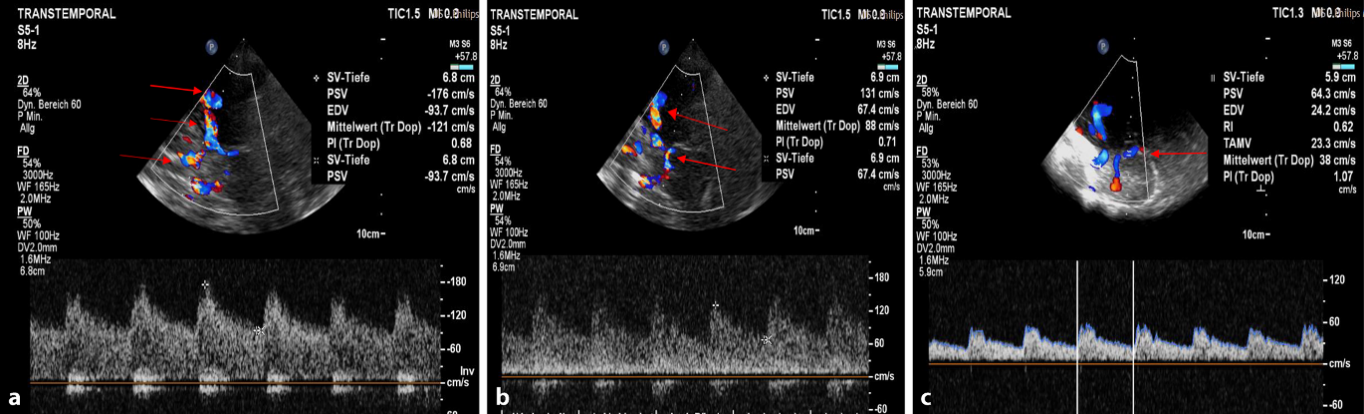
Transkranielle Duplexsonographie: a, b intrakranielle Flussbeschleunigungen in der A. cerebri media links und rechts sowie in der A. cerebri anterior und posterior links (*Pfeile*); c transkranielle duplexsonographische Verlaufskontrolle nach 3 Wochen mit lediglich residualer leichter Flussbeschleunigung in der A. cerebri media links (*Pfeile*) und ansonsten normalen Flüssen in der A. cerebri anterior und posterior links
